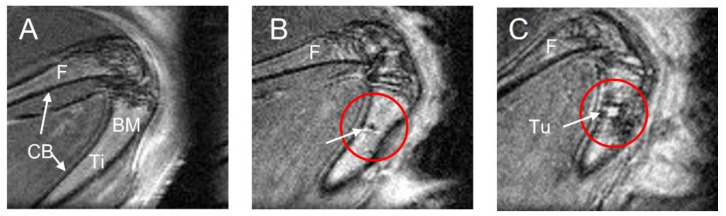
Tibia and tumor phenotype in MRI. (A) Femur (F) and tibia (Ti) with tumor-free bone marrow cavity (BM) and cortical bone (CB); (B) tibia with a possible sign of the injection as scar tissue or blood marked with an arrow in a red circle; (C) intratibial tumor (Tu) with high signal intensity, possibly necrosis/inflammation, marked with an arrow in a red circle.
